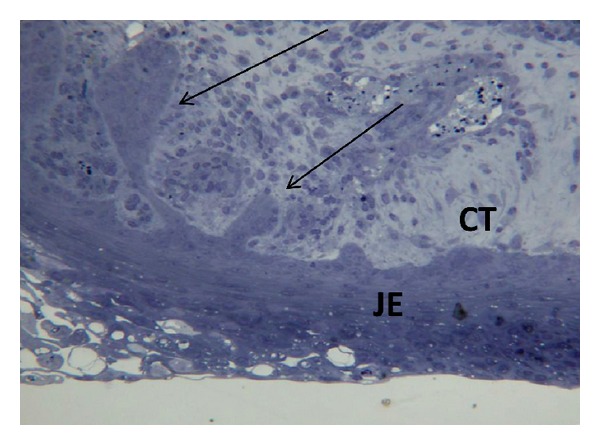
Primary JE culture treated with *A. actinomycetemcomitans *LPS shows migration of the epithelium into CT (arrows).
